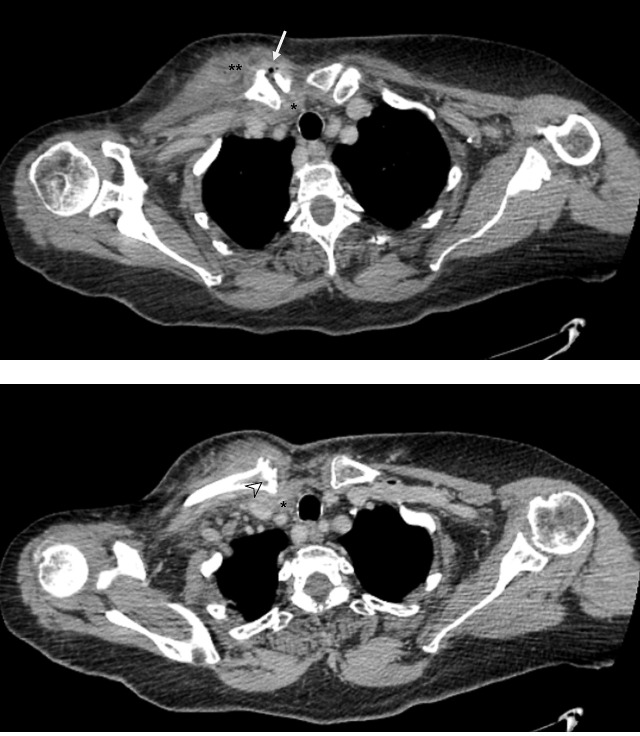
CT scan of the chest demonstrating signs of septic arthritis of the right SCJ, with articular space widening with gas formation, integrity
